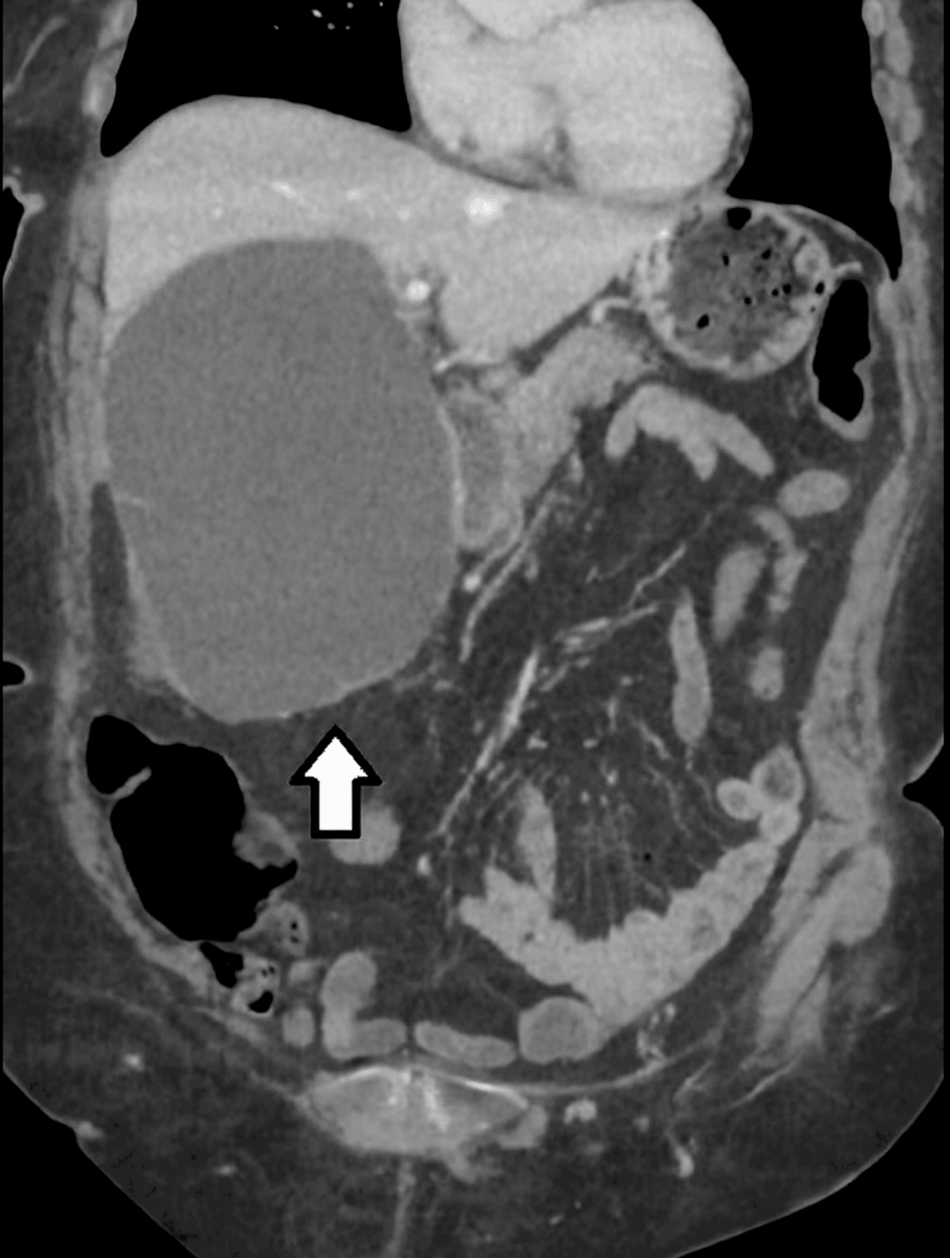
Coronal CT image The white arrow indicates reaccumulation of homogeneous, low-attenuation fluid within the cavity of the ruptured hepatic cyst, consistent with sterile fluid collection.
